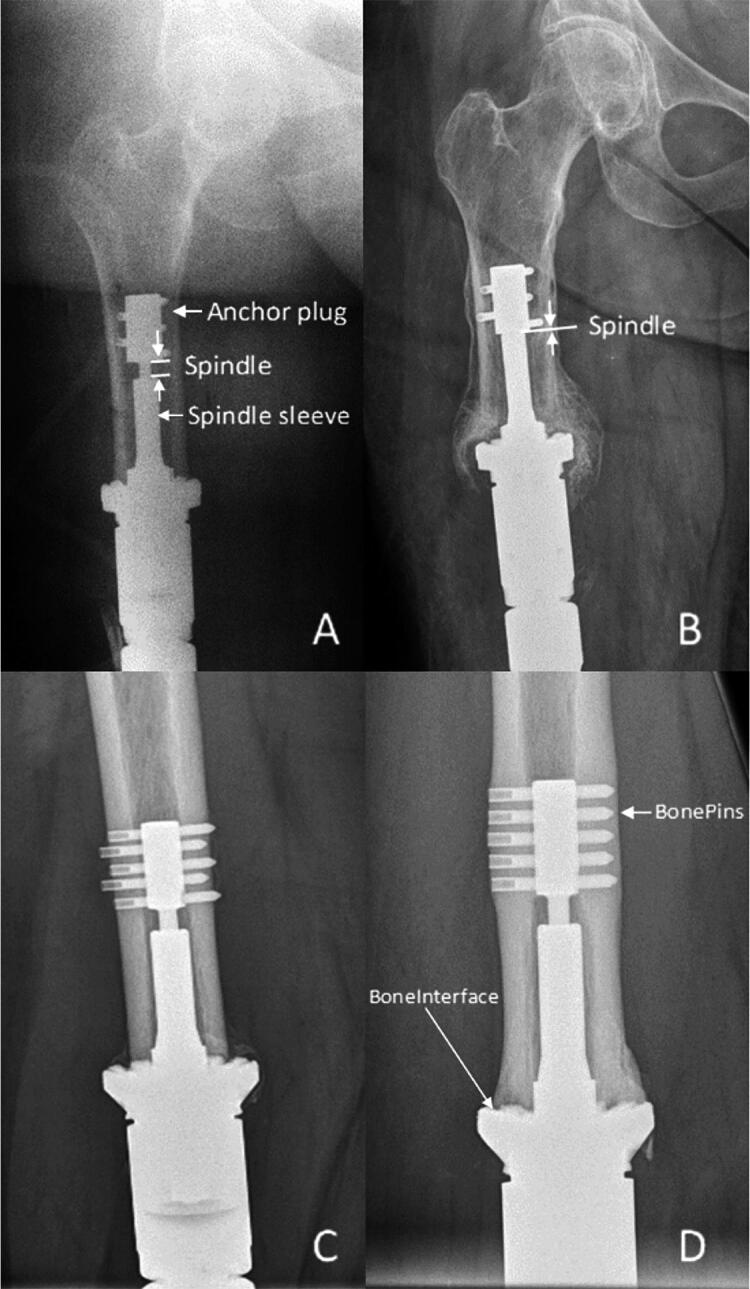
Radiographic parameters used to construct the fast and frugal tree model.
